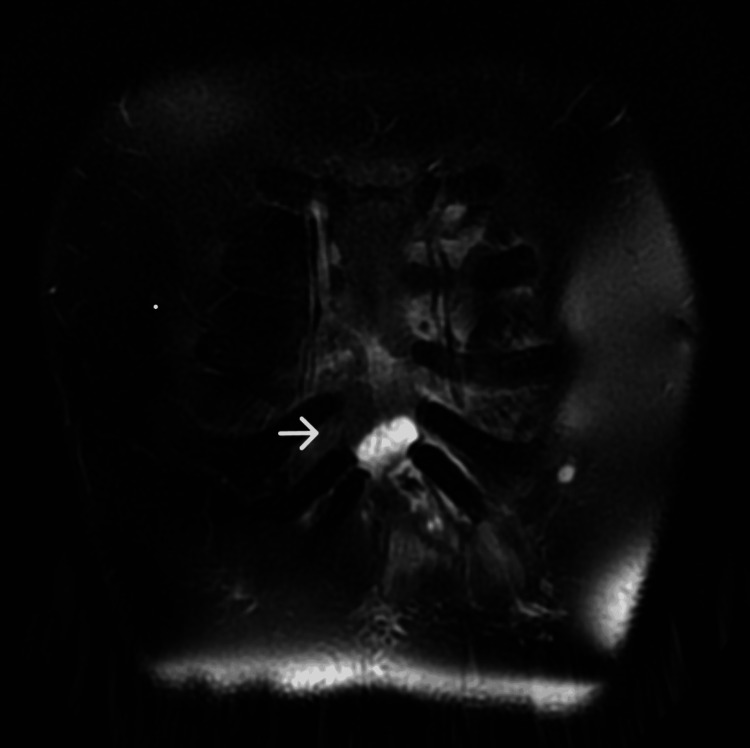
Magnetic resonance imaging with fat-suppressed T2-weighted image showing high intensity in the inferior sternum (arrow)
